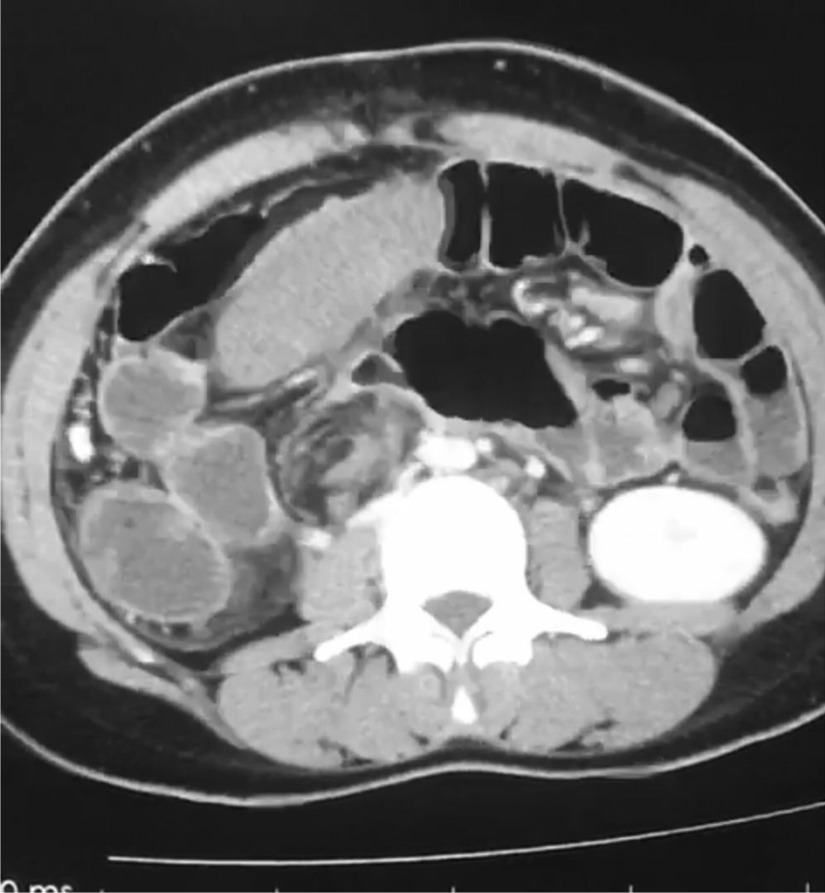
An axial section of the CT scan showing a "whirl sign" (pathognomonic for pedicle torsion) of the splenic pedicle
